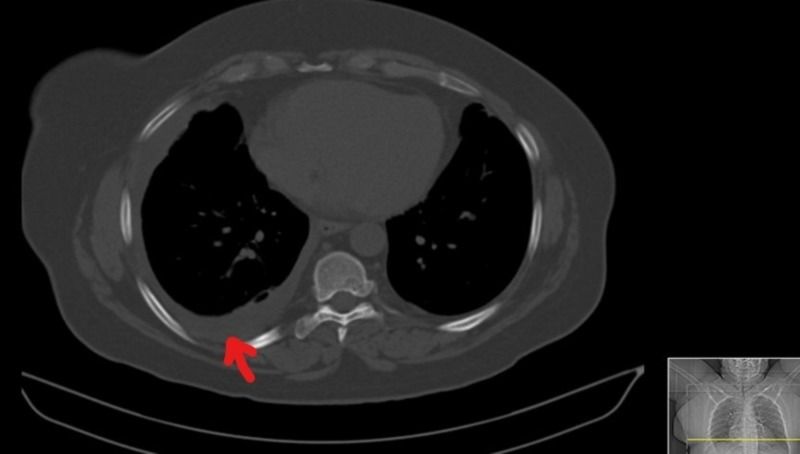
Thoracic computed tomography (CT) scan from day 1
